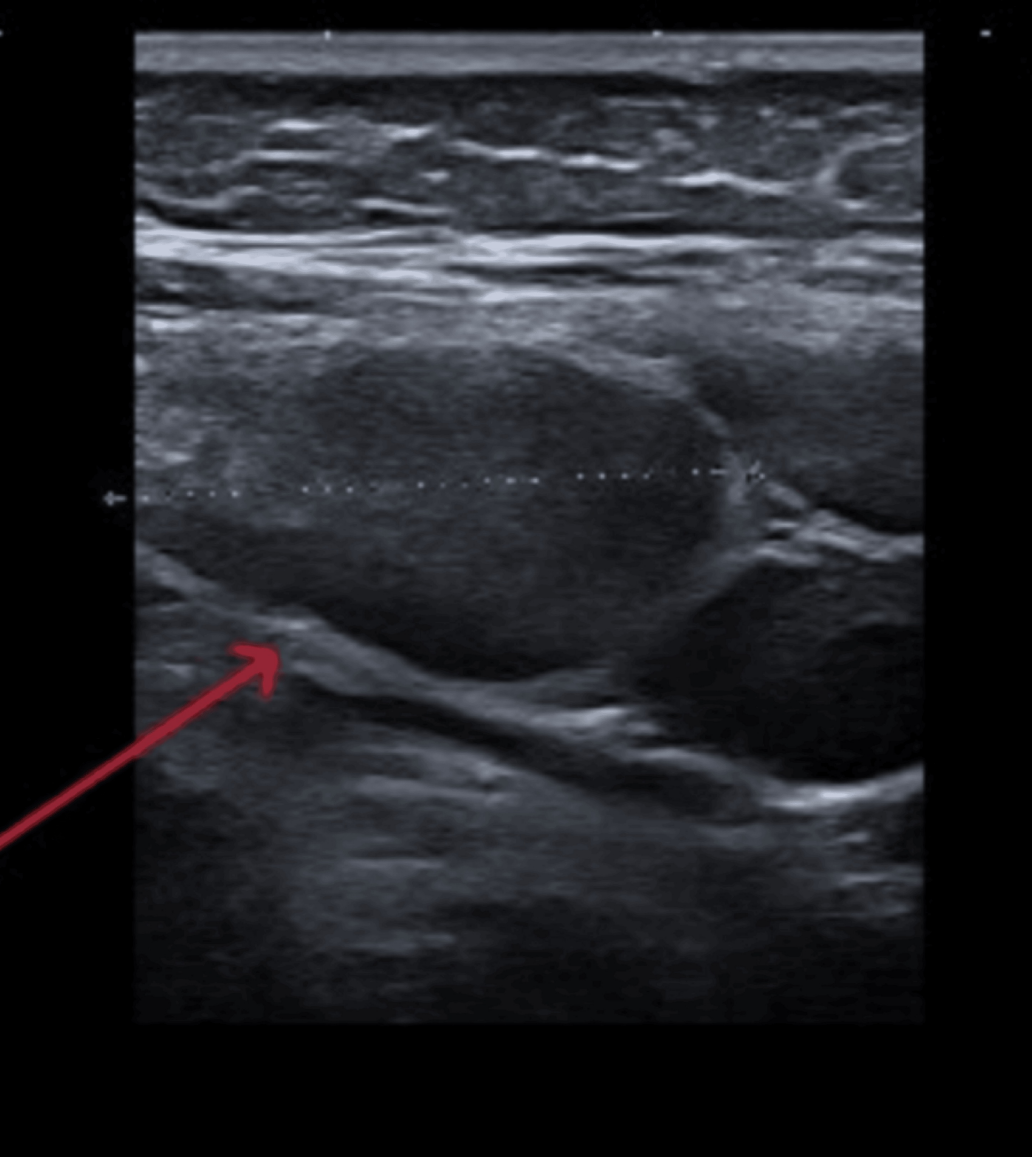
Ultrasound image of the neck showing right-sided enlarged lymph nodes (arrow)
